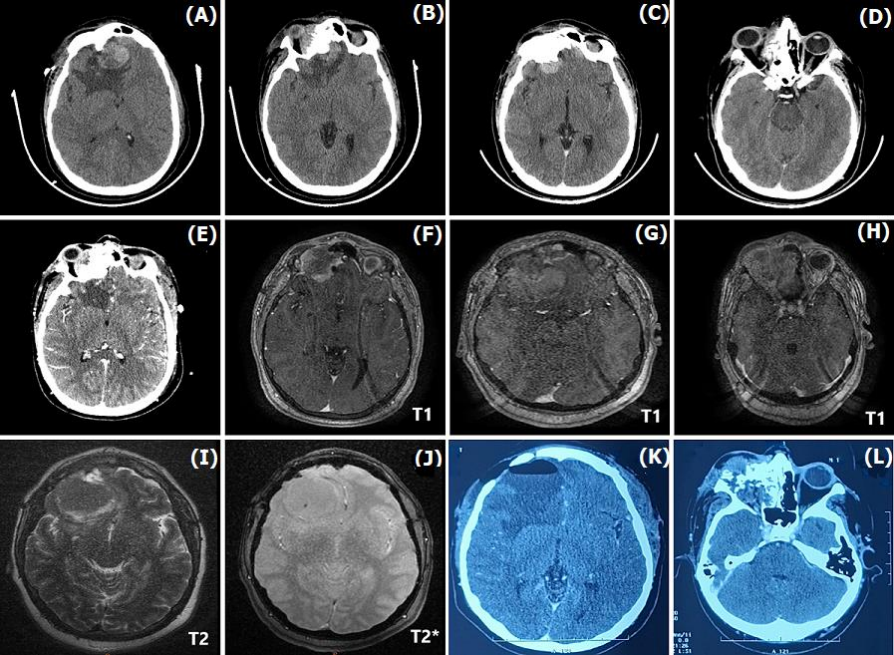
A, B, C, D, E, F, G, H, I, J, K, L) evolution of a right frontal extra-axial mass with recurrences and extensions, confirmed by computed tomography and magnetic resonance imaging
